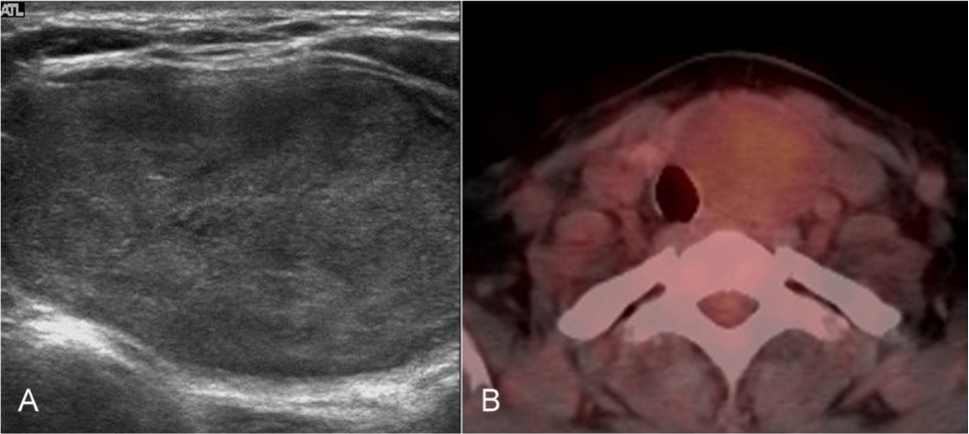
Images of follicular adenoma. a On ultrasonography, there is an 8-cm, markedly hypoechoic nodule. b Mildly increased ^18^F-FDG uptake in the left lobe on ^18^F-FDG PET/CT (SUVmax = 2.10)
